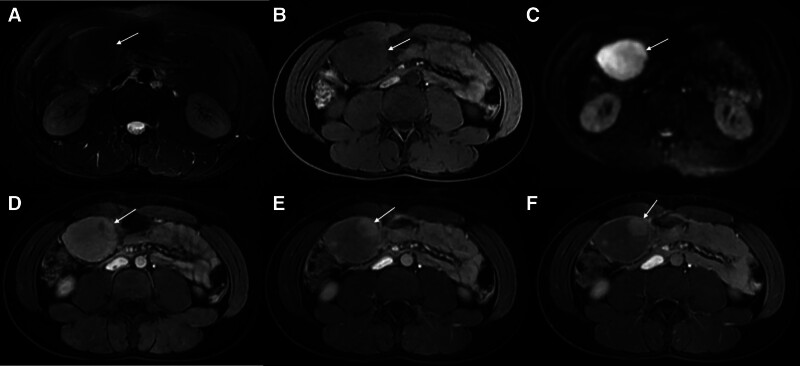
Magnetic resonance images image of gastric FDCS. (A) Axial T2-weighted MRI showed well-defined, hypointense mass (white arrow) in right mid-abdomen. (B) The mass was isointense on axial T1-weighted MRI. (C) On axial DWI, the mass was obviously hyperintense. (D–F) Axial T1-weighted contrast-enhanced MR images in arterial (D) portal (E) and delayed (F) phases showed uneven strengthening. DWI = diffusion weighted imaging, FDCS = follicular dendritic cell sarcoma, MRI = magnetic resonance images.
